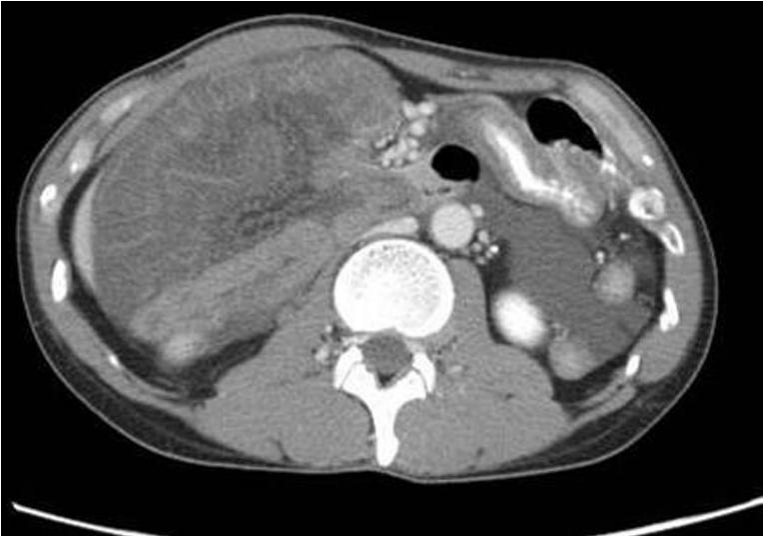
Pre-operative enhanced abdominal multidetector-row computed tomography shows intestinal cluster in the right lateral abdominal cavity. The intestinal wall is edematous and not well-enhanced.
